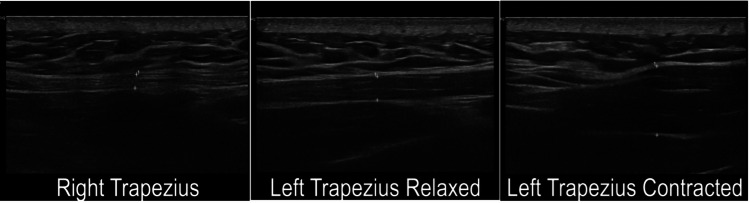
Ultrasound (US) images of the patient's trapezii; only one image of the smaller, atrophied right trapezius was included, as there was no change with volitional contraction. The left trapezius shows normal growth in size when contracted.
